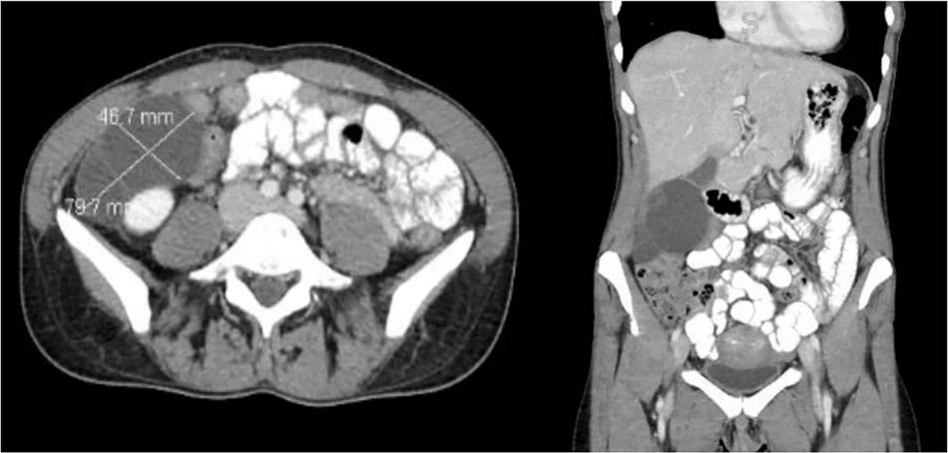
CT showing cystic structure abutting the right kidney, liver, and gallbladder.
